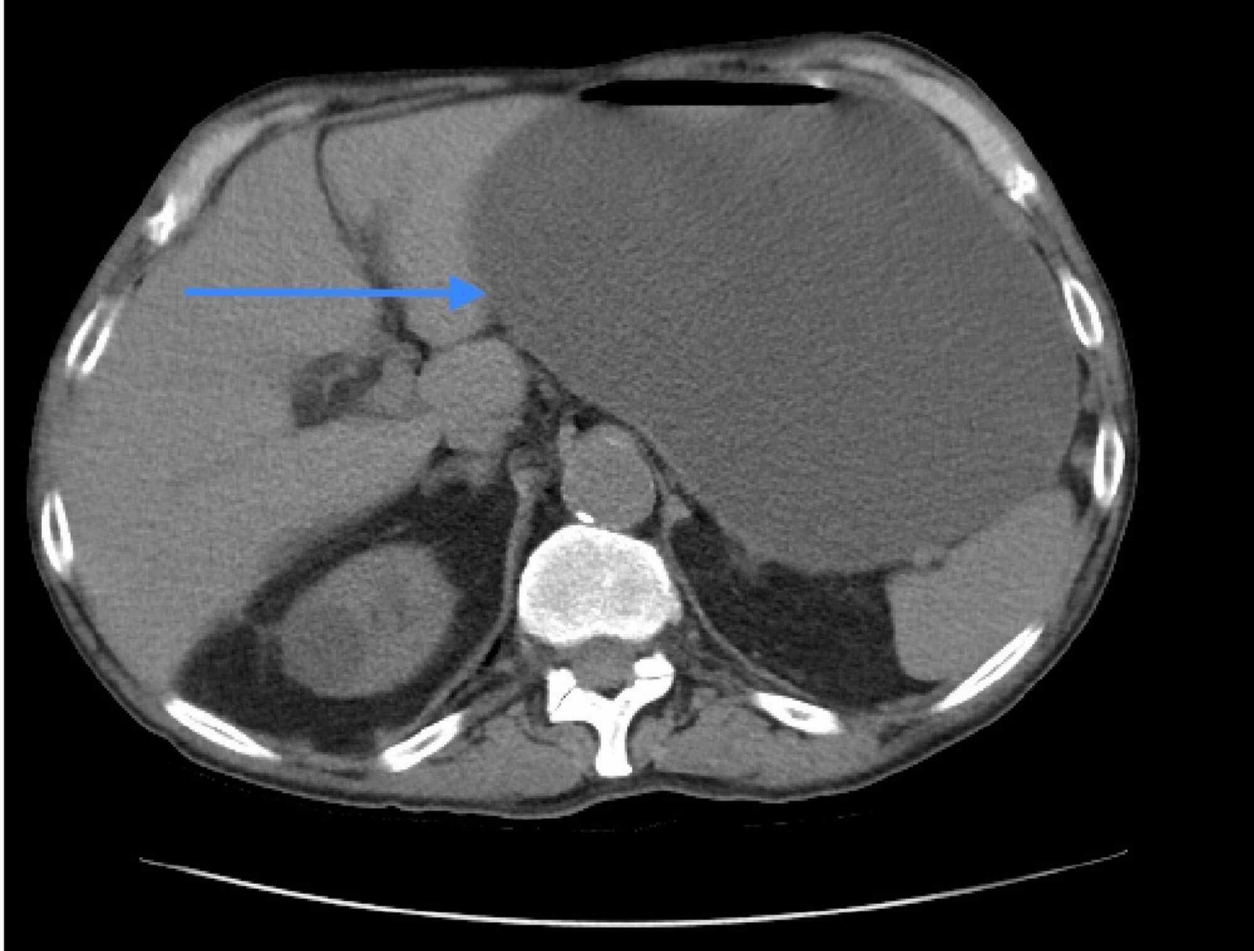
Axial view abdominal CT scan without IV contrast showing a markedly distended stomach
